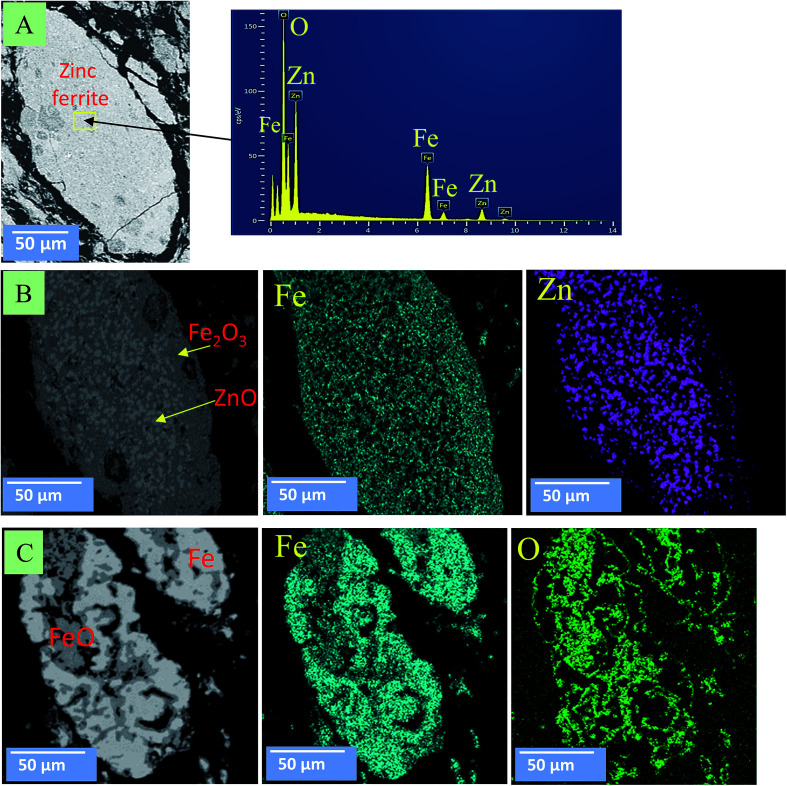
SEM images for zinc ferrite under the CT reaction. (A) At 850 °C; (B) at 950 °C; (C) at 1050 °C.
